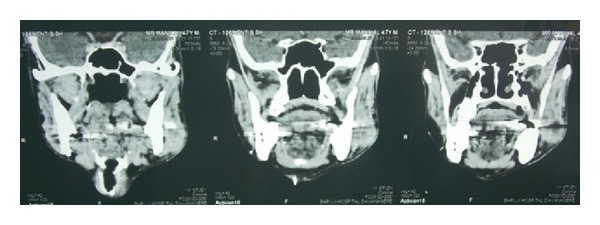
CT scan reveals cystic lesion in masseteric space involving the ramus of the left mandible with lytic and sclerotic area.
